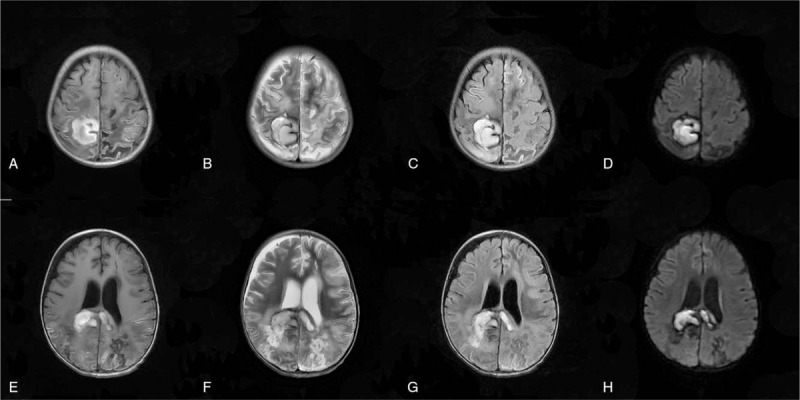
Head MRI on day 35 after surgery. Note: A and E are T1WI, B1 and B2 are T2WI, B and F are FLAIR, C and G are FLAIR, and D and H are DWI. DWI = diffusion-weighted imaging, FLAIR = fluid attenuated inversion recovery, MRI = magnetic resonance imaging, T1WI = T1 weighted image, T2WI = T2 weighted image.
